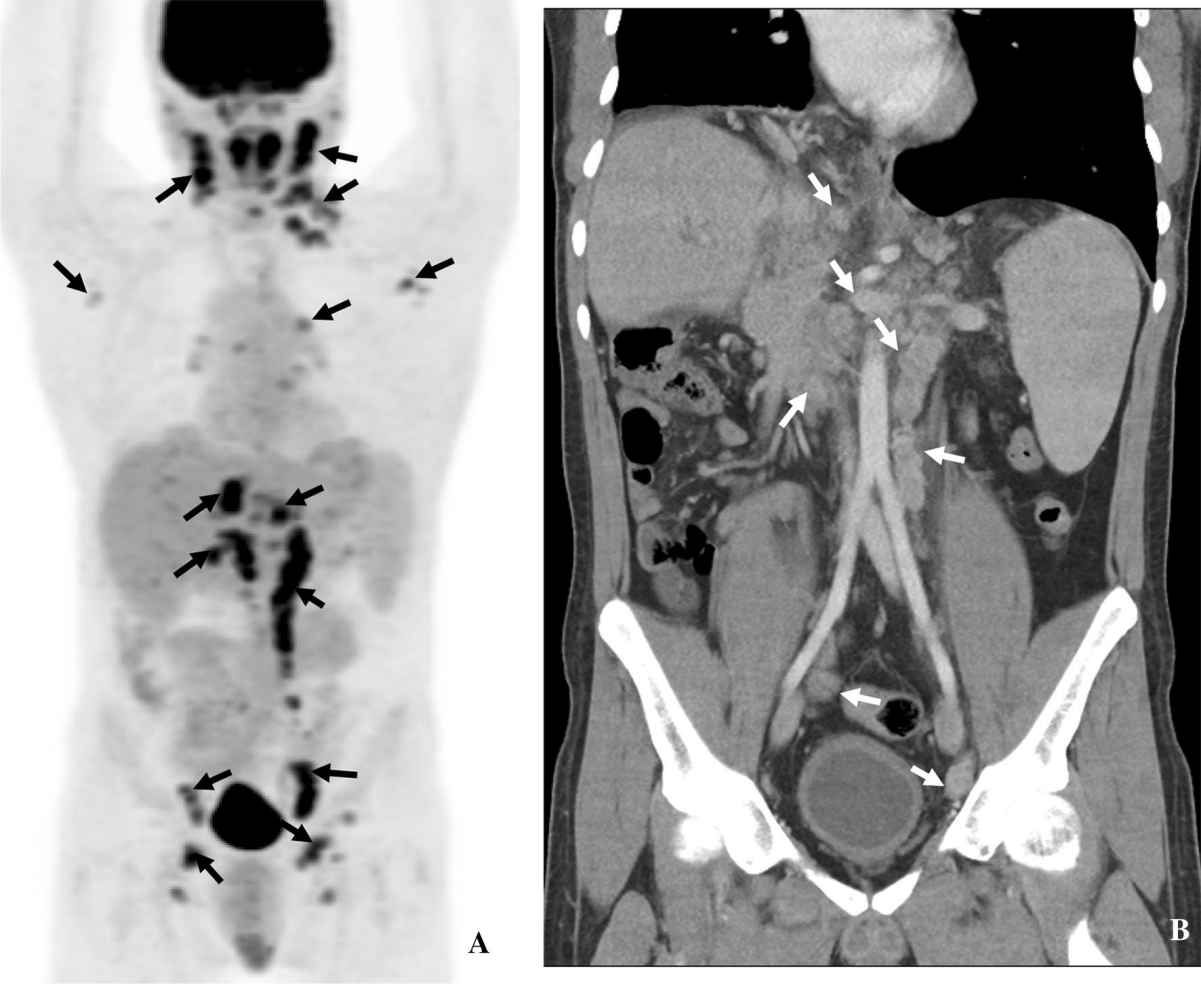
31-year-old male with KS and history of immunosuppression for liver transplant secondary to biliary atresia. A PET showing ^18^F-FDG uptake in multi-compartment lymphadenopathy (arrows). B Coronal CT showing multicompartment lymphadenopathy in the abdomen and pelvis (arrows)
